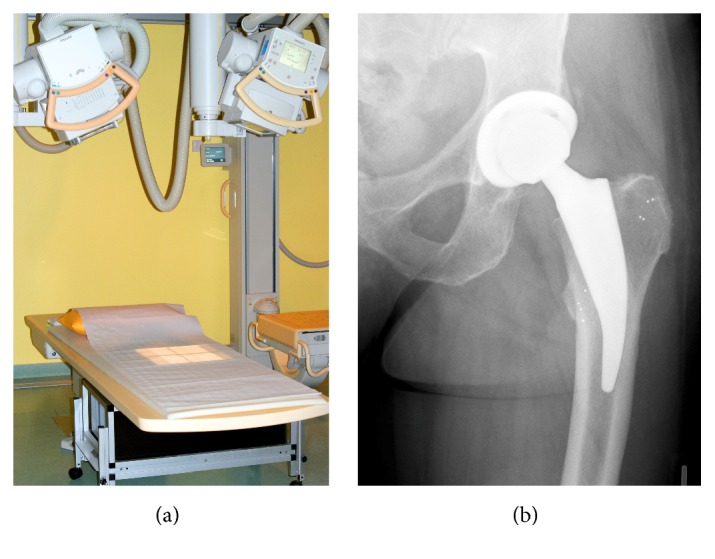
Uniplanar RSA measurement setup. (a) The X-ray tubes were each oriented at a 20° angle to the horizontal and the film to focus distance was 160 cm. The carbon calibration box was under the examination table. (b) Implant migration was calculated by rigid body kinematics using up to ten tantalum beads, which were inserted into the bone surrounding the implant and serving as fixed reference.
